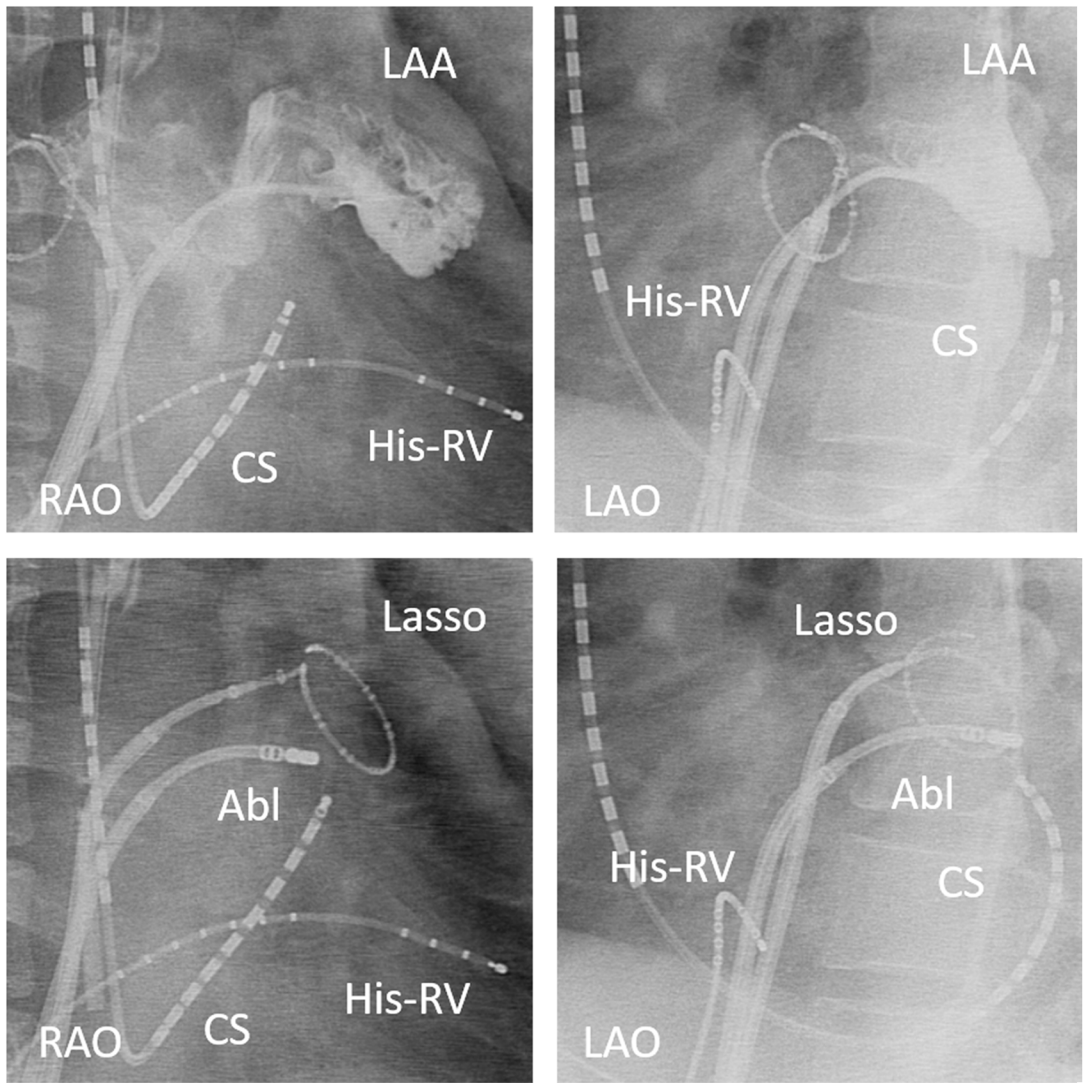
Angiography of the left atrial appendage (LAA) showing the boundary between the LAA and lateral free wall (upper panel) Radiography showing the circular mapping catheter in the left atrial appendage, and right anterior oblique (RAO) and left anterior oblique (LAO) views of the catheter position during the mitral isthmus ablation (lower panel). The ablation was started with the ablation catheter located at the earliest activation site during CS pacing. A steerable sheath was used to facilitate the catheter manipulation.
